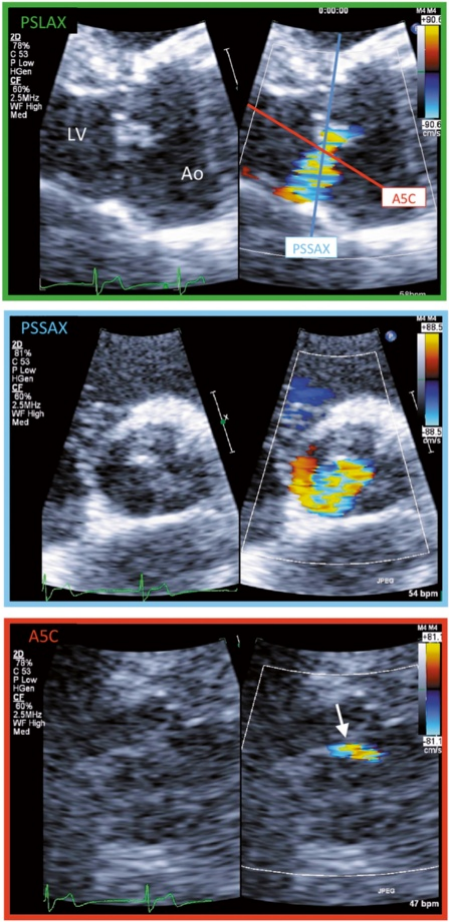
Analysis of two-dimensional color flow imaging in a patient with a bicuspid aortic valve and an eccentric aortic regurgitation (AR) jet. Parasternal long-axis view (top). After selecting the best frame for AR jet visualization, the three components of AR (the proximal flow convergence, the vena contracta, and the distal expansion of the regurgitant jet spray) were identified. Standard parasternal short-axis view (middle). This image plane was almost parallel to the AR jet, and two-dimensional vena contracta area (2DVCA) measurement was not indicated. Apical five-chamber view (bottom). This image plane was almost perpendicular to the long axis of the AR jet. The smallest area between the proximal flow convergence and distal regurgitant jet spray was measured as 2DVCA (arrow). PSLAX, parasternal long-axis view; PSSAX, parasternal short-axis view; A5C, apical five-chamber view; Ao, aorta; LV, left ventricle.
